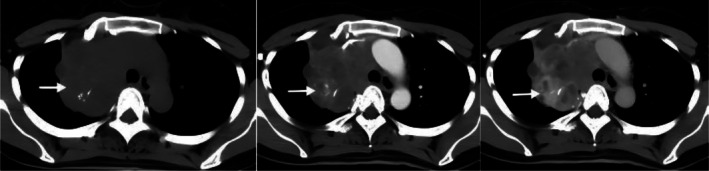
Initial chest CT. (1) Axial noncontrast image shows a large mass in the right upper lobe with speckled calcifications (→). (2) Arterial phase shows heterogeneous enhancement (→). (3) Venous phase shows progressive enhancement (→).
